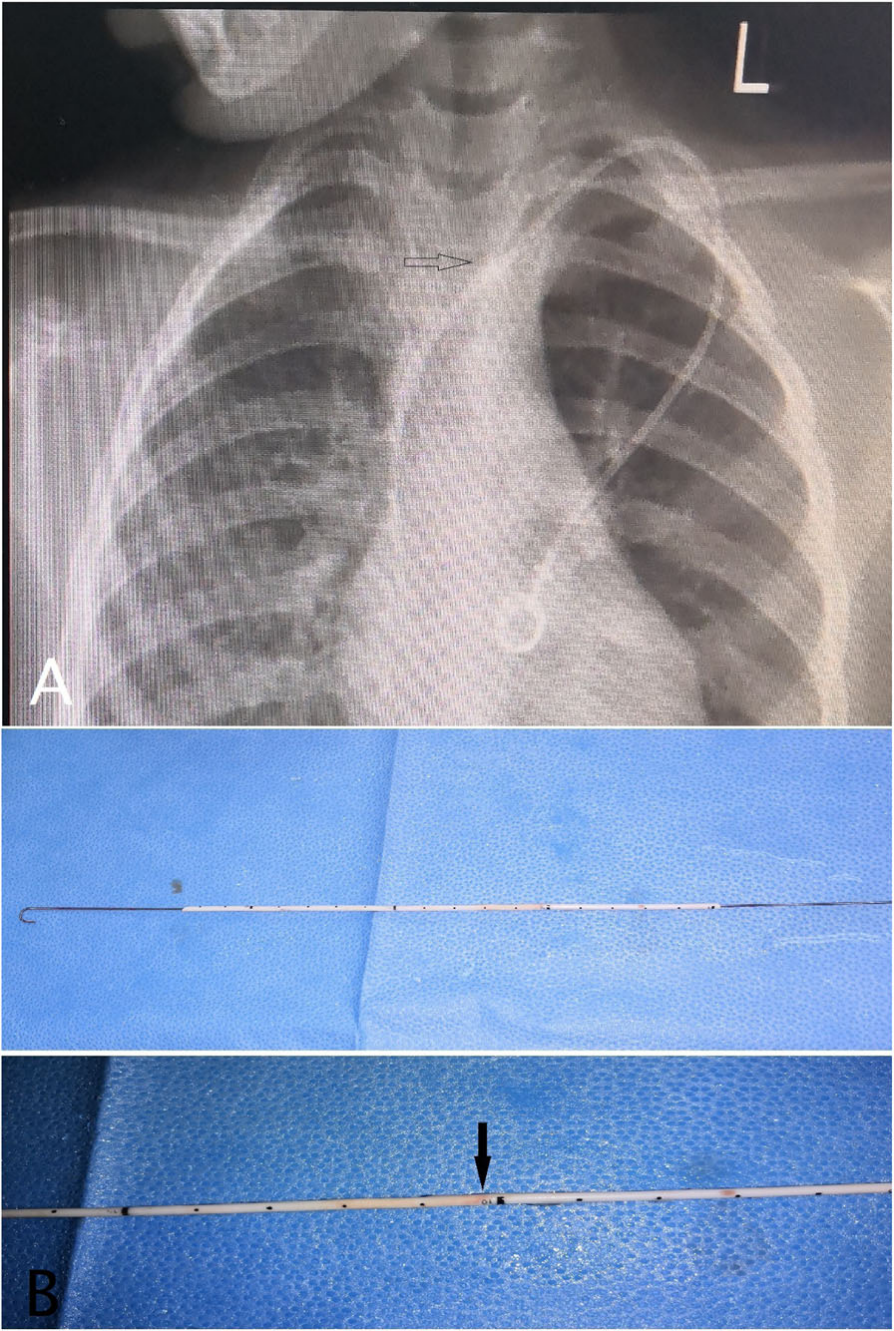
a Chest X-ray showing thickening of the catheter at the subclavian vein (black arrow).The catheter was stuck and removed by inserting a good-sized guidewire in it and pull it. b The catheter after removal. The black arrow shows the site of the adhesion on the catheter
